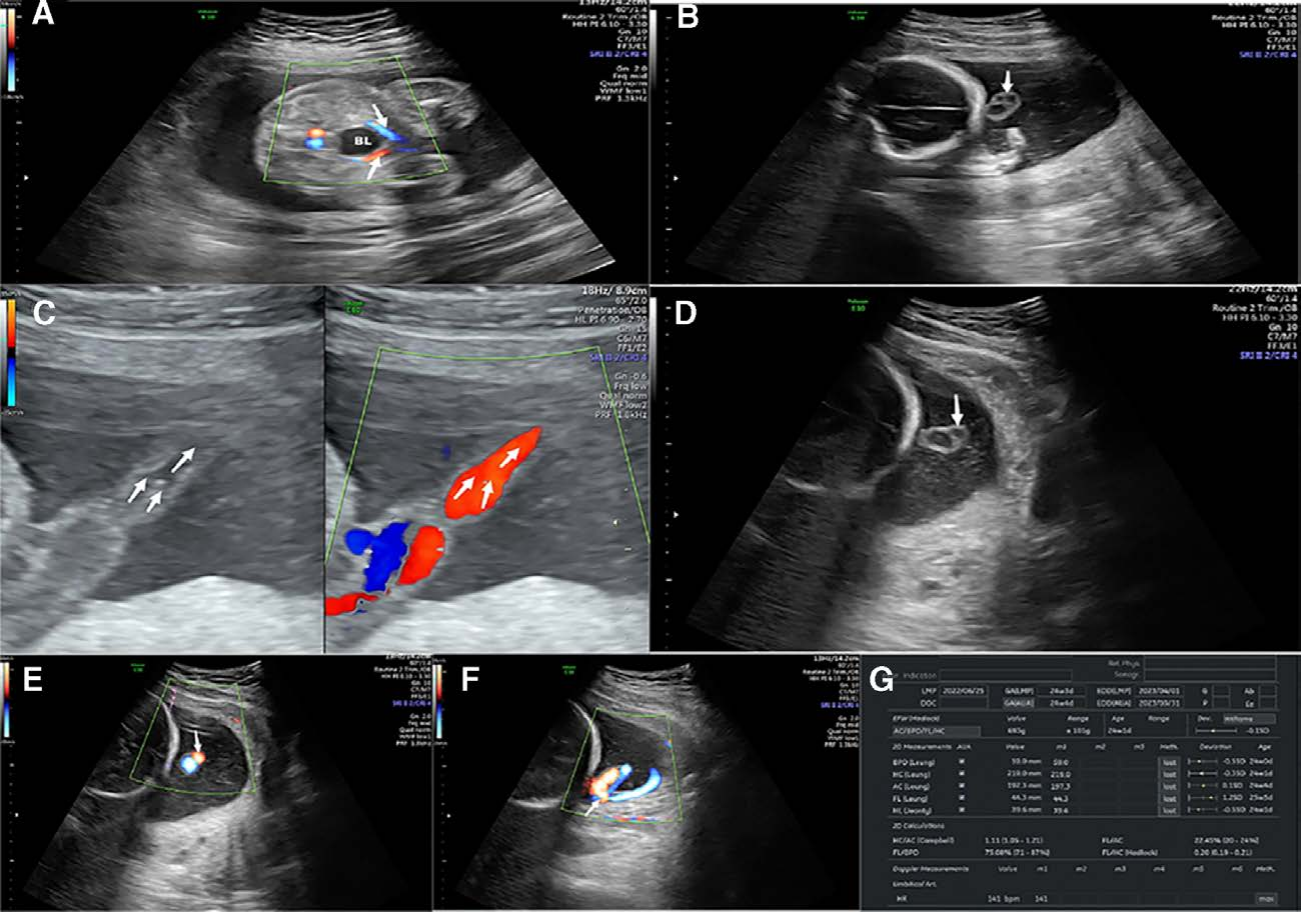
(A) Two umbilical arteries derived from the 2 arteries of the urinary bladder. (B) Normal bilateral umbilical arteries are shown in the free segment. (C) Two umbilical arteries converging into a single umbilical artery. (D) A single umbilical artery in 2 dimension. (E) A single umbilical artery shown on CDFI. (F) The umbilical cord was inserted into the amniotic sac. (G) Table of fetal biological measurements. CDFI = Color Doppler Flow Imaging.
